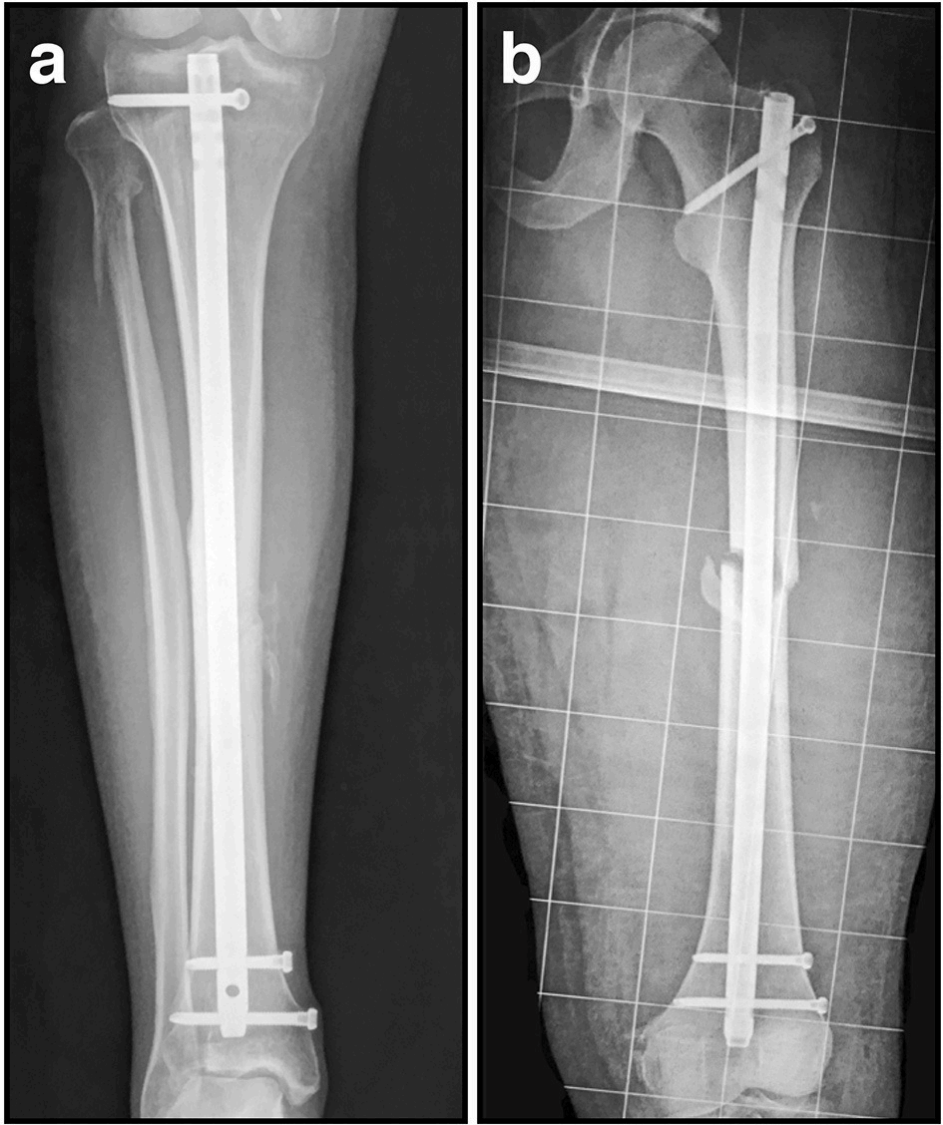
Radiographic intramedullary nail images. (A) Statically locked tibial nail to stabilize a mid-diaphyseal fracture; (B) Statically locked femoral nail to stabilize a mid-diaphyseal short oblique fracture.
